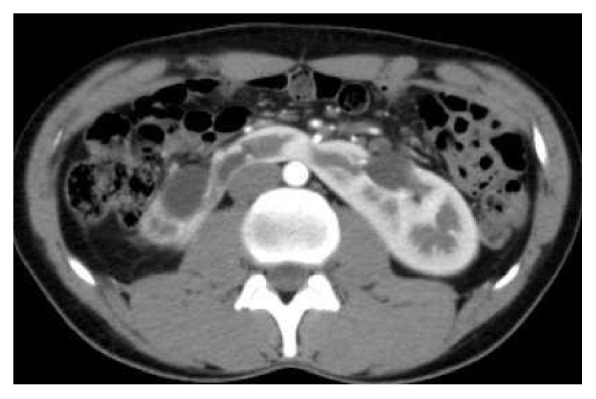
Initial preoperative CE-CT scan (axial section) showing horseshoe kidney with the fusion of the lower poles to the left of aorta.
